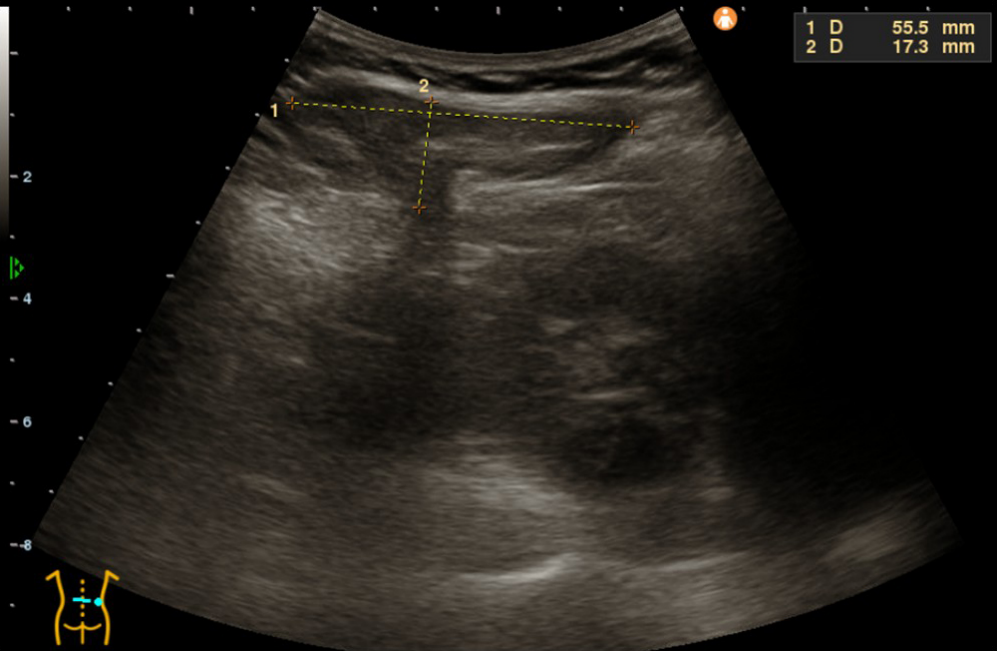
Ultrasonographic image showing a hyperechoic mass (measuring 55.5 mm × 17.3 mm) protruding through a fascial defect in the right superior lumbar region, consistent with herniated fat.
